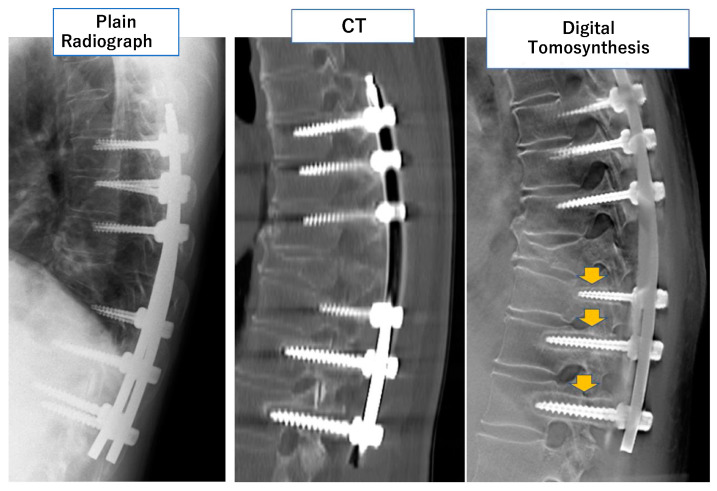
Comparison among plain radiography, computed tomography (CT), and digital tomosynthesis (DT) in an evaluation of pedicle screw loosening after spinal instrumentation. In DT, radiolucent areas around PS were more evident than in images obtained by the two other modalities (arrows).
